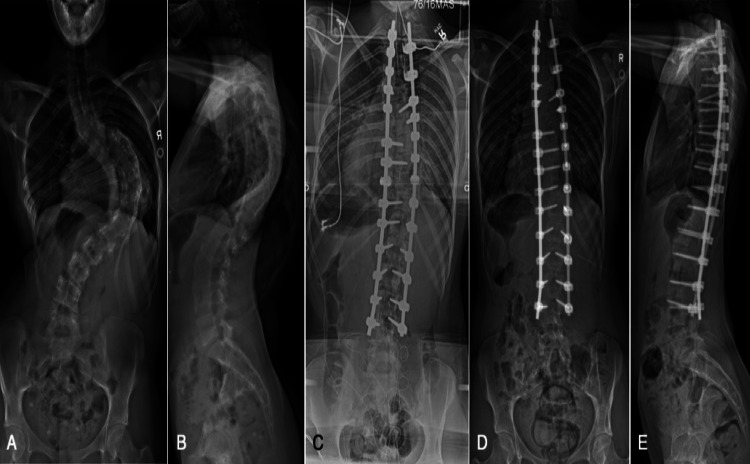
The pre- and post-operative radiographs observed in Case 2. Preoperative standing posteroanterior (A) and lateral (B) radiographs of a 16-year-old female with a maximum coronal Cobb of 110° with apex at T9-10 and a sagittal Cobb of 65° with apex at T9. Intraoperative posteroanterior radiograph (C) demonstrating reduction of the coronal deformity with a construct from T2-L4. Two-month postoperative standing posteroanterior (D) and lateral (E) radiographs.
